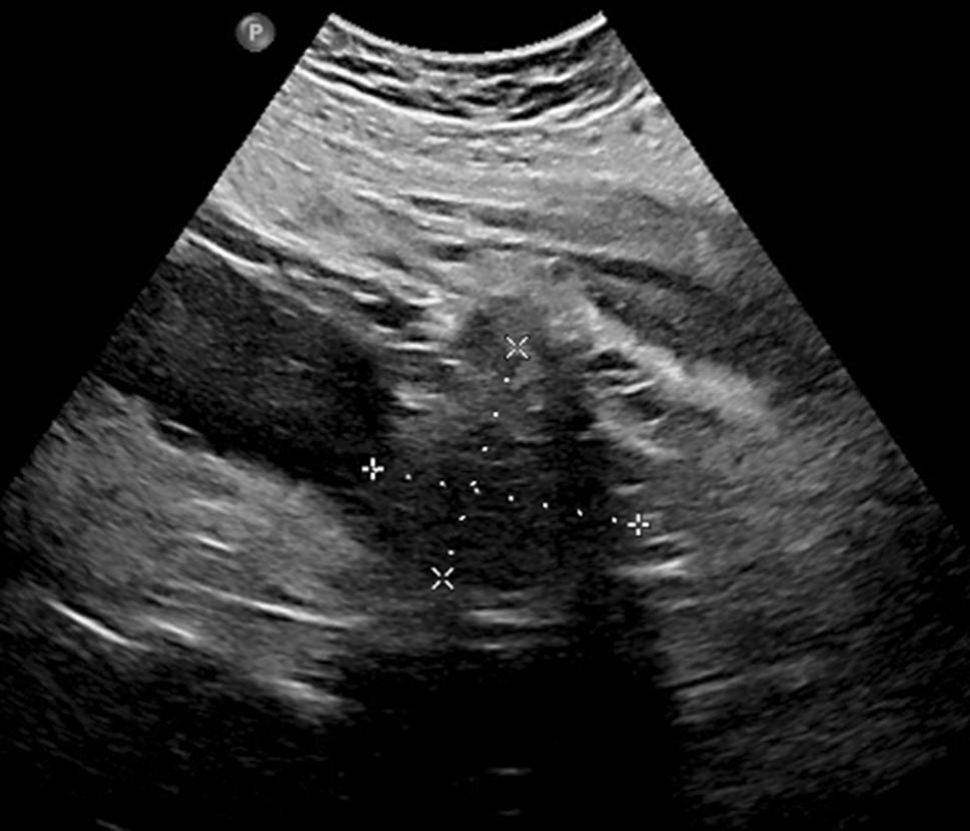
Sonography in 26 + 2 weeks of gestation: psoas abscess with 5 × 5 cm
